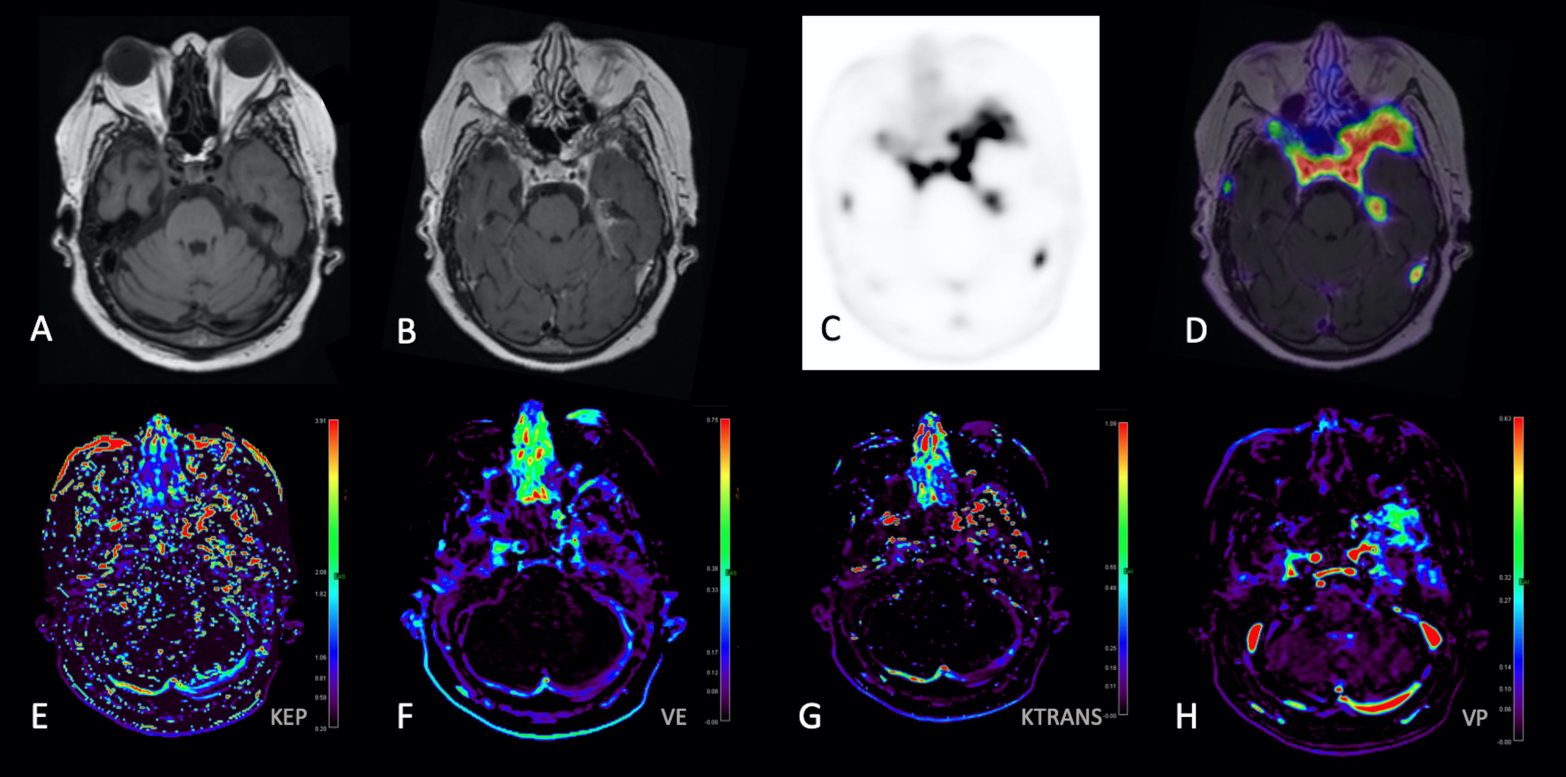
68-Gallium-DOTATATE PET and DCE Perfusion MRI images in a 68-year-old woman with WHO-1 left anterior temporal convexity meningioma post prior surgical resection and RT. Axial T1-weighted (A), gadolinium-enhanced axial T1-weighted (B), static 68-Gallium-DOTATATE PET image (C), axial PET/MR fusion image (D), Kep parametric map (E), Ve parametric map (F), Ktrans parametric map (G), and Vp parametric map demonstrates extra-axial homogeneously enhancing soft tissue with corresponding 68-Gallium-DOTATATE avidity [(D), with SUV measured along the left anterior temporal convexity] and abnormal perfusion parametric maps (E–H), corresponding to region of suspected residual/recurrent meningioma.
